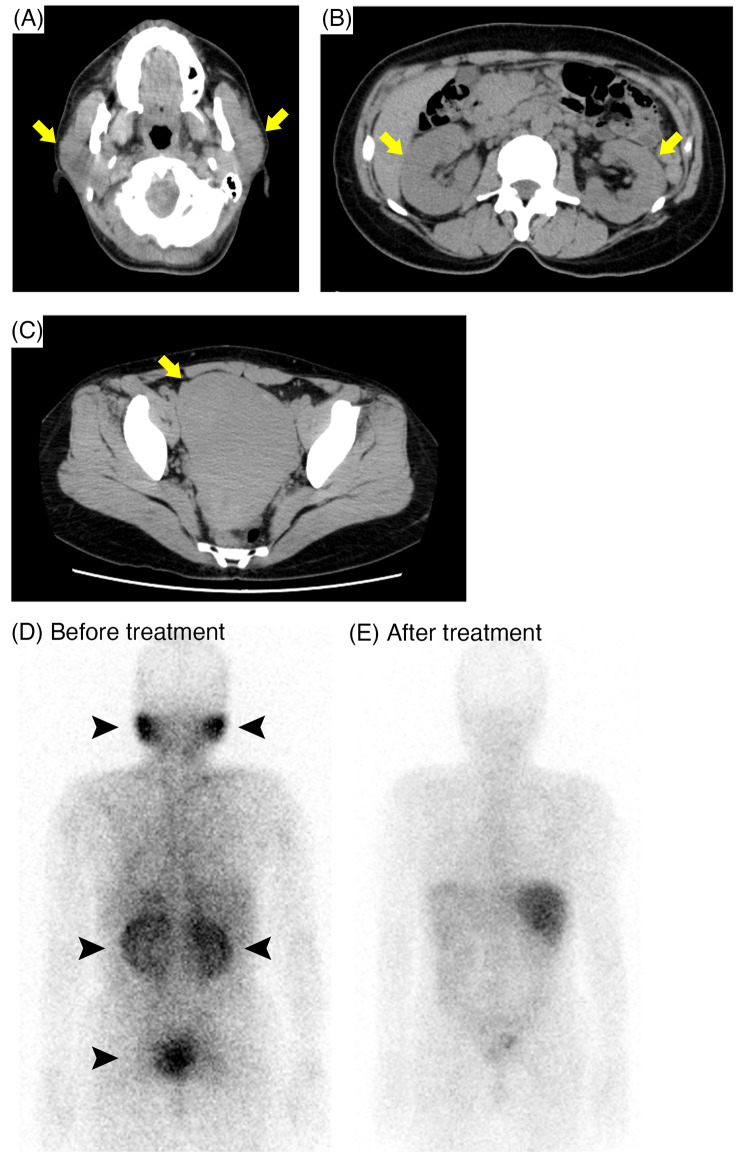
Findings of Computed tomography (CT) scan and Ga scintigraphy. (A, B, C) CT scan showed swelling of parotid glands (A), bilateral kidneys (B), and uterus (C, arrows). (D) Ga scintigraphy before treatment showed remarkable accumulation in these organs (arrowheads). (E) After the treatment, the accumulation has disappeared
